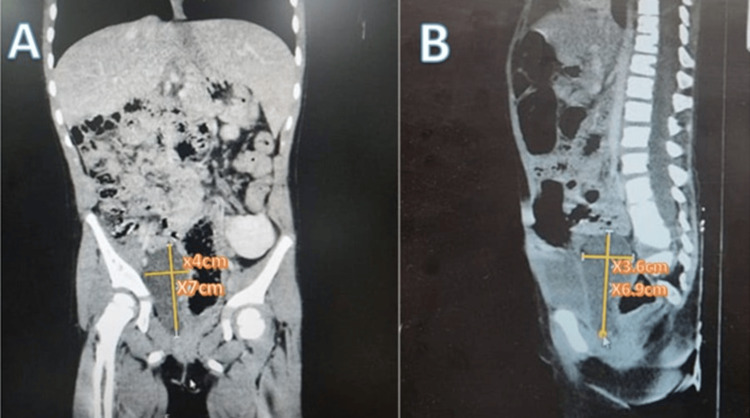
Computed tomography (CT). (A) Coronal view reveals a non-enhancing, well-defined cyst in the right pelvic cavity, measuring approximately 7 cm long and 4 cm wide. Additionally, a beaver tail liver was noted, along with mild scoliosis. No other significant issues were observed. (B) In the sagittal view, the depth of the cyst is measured as 3.6 cm, with the upper part located presacrally and the lower part posterior to the urinary bladder in the right pararectal space. No calcifications, septations, or soft tissue are present inside the cyst, and there is no wall enhancement.
